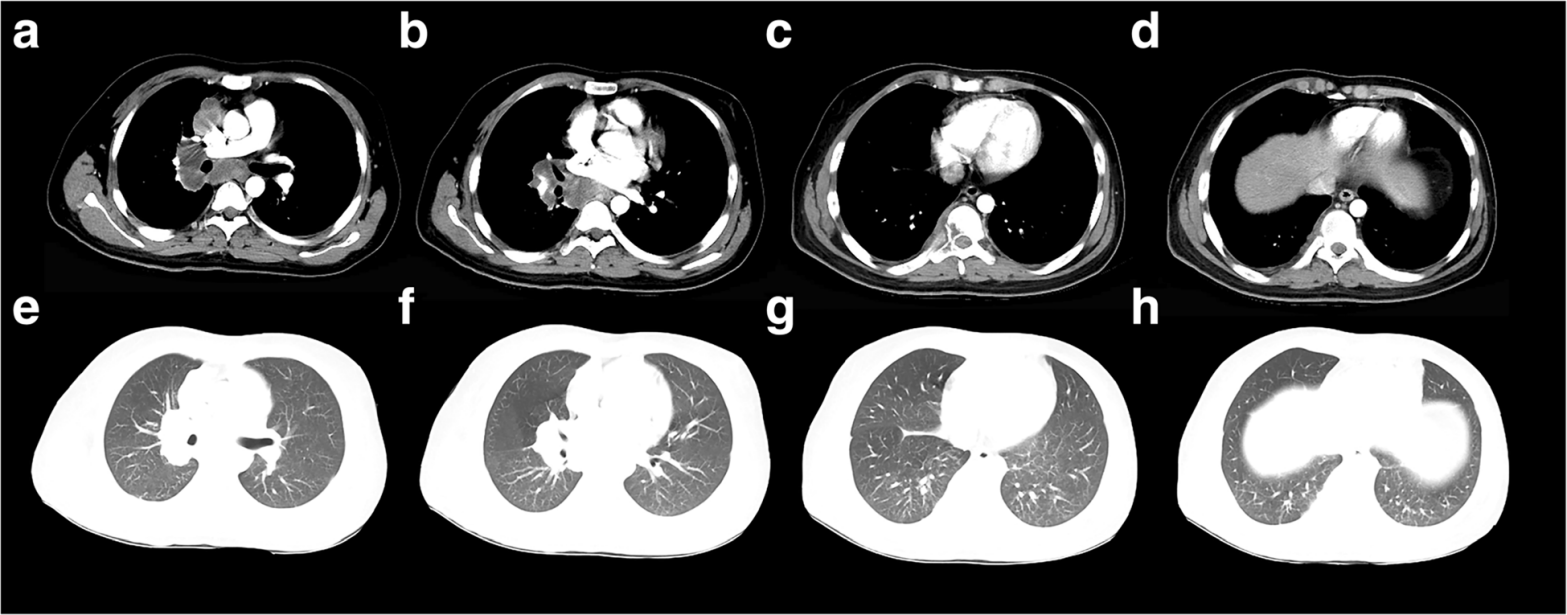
Representative slices of enhanced chest CT images showed a, b multiple enlarged lymph nodes in the mediastinal and right hilus of lung, c, d bone destruction and soft tissue density mass in the body and appendage of T8, and right eighth rib head and e-h chronic inflammation changes of the lungs
